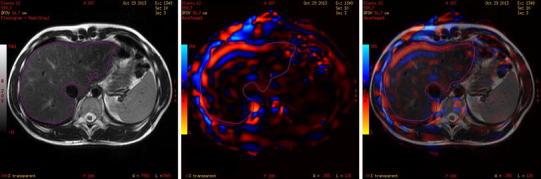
Single cross section of the liver. Whole-liver stiffness was calculated as a mean of such ROIs drawn on all axial slices of the liver. The ROI is presented on T2-weighted image (*left*), elastogram (*middle*), and fused image of T2WI and elastogram (*right*).
